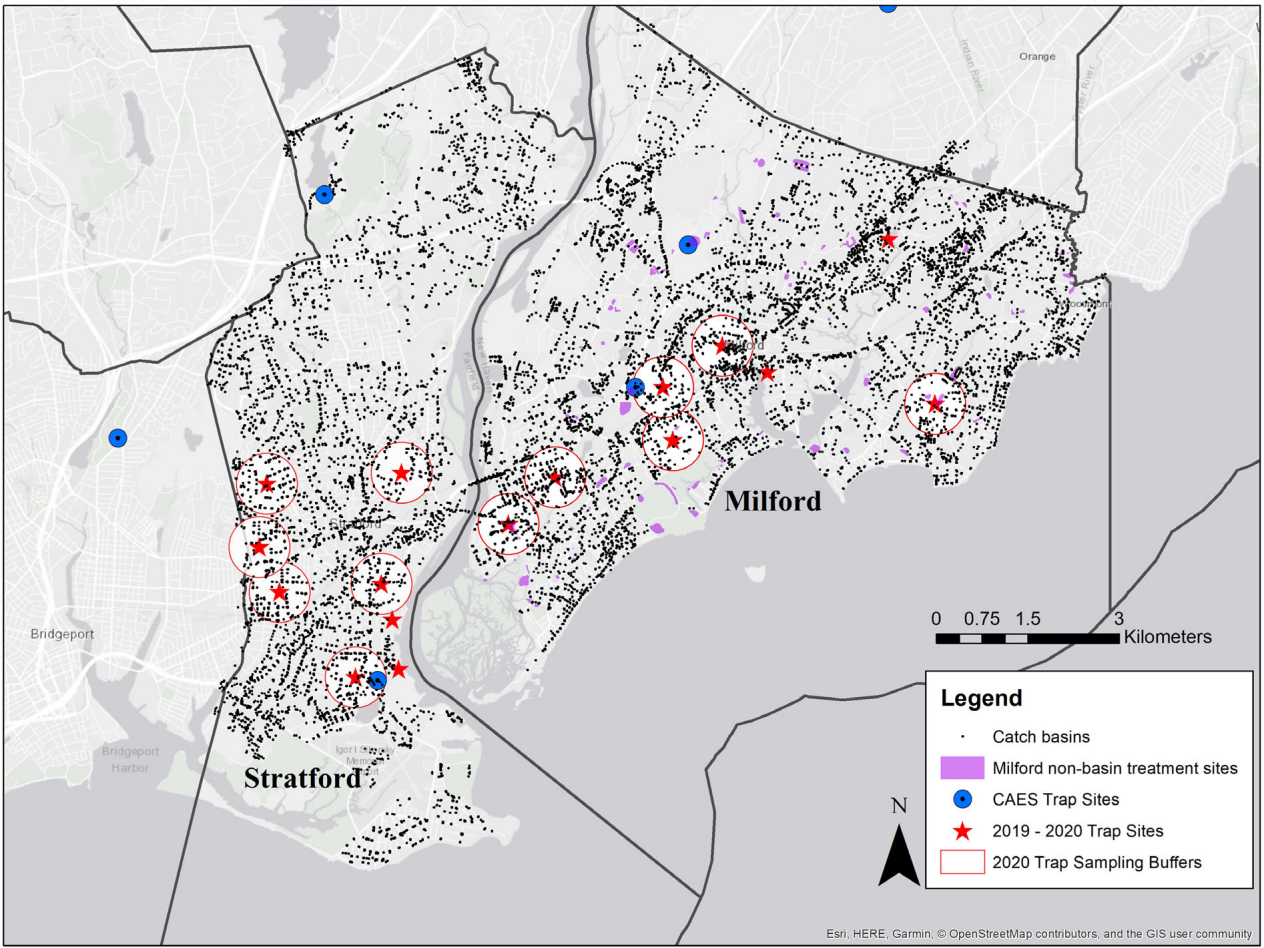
Map of surveillance and catch basin sampling sites in Milford and Stratford, CT 2019–2020. Red stars, adult mosquito and West Nile virus surveillance sites; white with red border circles, 500 m sampling buffer instituted in 2020; small black points, catch basin locations; purple regions, nonbasin larvicide treatment areas in Stratford; solid black lines, each townʼs political boundary with town names listed in bold, black text. The Connecticut Agricultural Experiment Stationʼs statewide mosquito and arbovirus surveillance locations in the region are shown for reference using blue circles with black dots. The figure was produced in ArcMap 10.5.1 (ESRI) using the OpenStreetsMap World Base and Reference maps.
